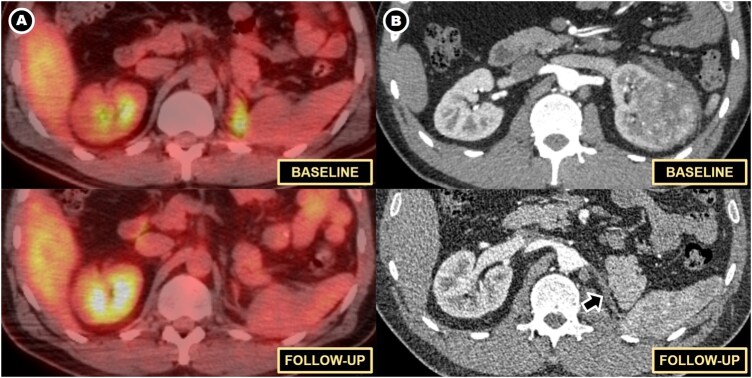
PET/CT and contrast-enhanced CT surveillance after corticosteroid therapy. (A) Axial fused PET/CT images through the left nephrectomy bed after IV administration of ^18^F-FDG, at baseline (top) and after 4 months of corticosteroid therapy (bottom), show marked metabolic regression of the previously hypermetabolic soft-tissue focus between the left diaphragmatic crus and spleen, consistent with treatment response of IgG4-related disease. No contralateral renal or retroperitoneal involvement. (B) Axial contrast-enhanced CT scan images through the left renal region at the corticomedullary phase, at preoperative baseline (top) and follow-up after 6 months of corticosteroid therapy (bottom), demonstrate no evidence of residual or recurrent renal malignancy. Only fibrotic postoperative changes (arrow) remain in the surgical bed on follow-up. No contralateral renal or retroperitoneal involvement.
